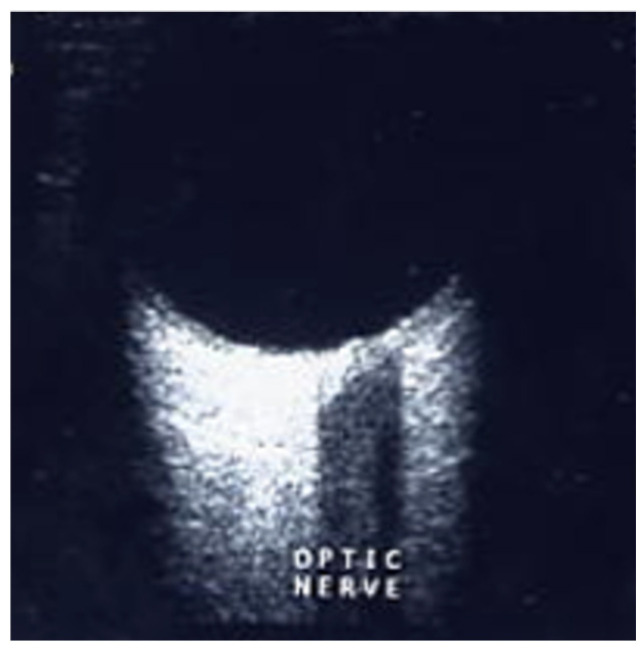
Posterior to the ocular globe, the optic nerve is easily detectable on ultrasound as a tubular hypoechoic structure.
